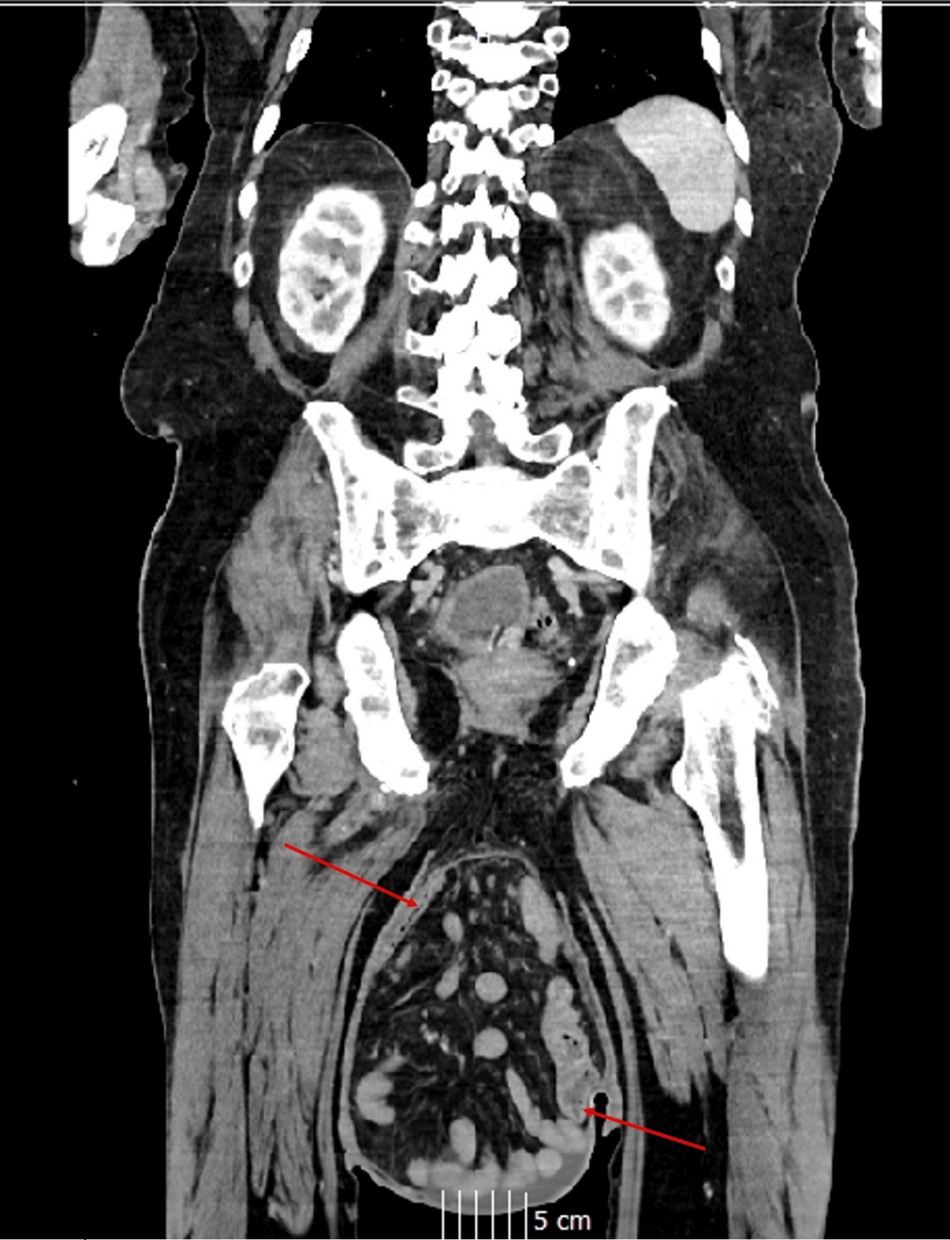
CT imaging of the abdomen and pelvis in a coronal view. Red arrows point to the inguinoscrotal hernial sac containing the stomach and large/small bowel. CT: computed tomography
